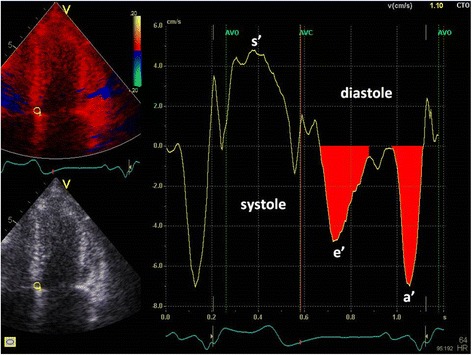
Image of colour tissue Doppler imaging with septal longitudinal displacement measurements depicted as area under the curve in early and late diastole. *s’, peak mitral annular systolic velocity; e’, peak early mitral annular diastolic velocity; a’, peak atrial mitral annular diastolic velocity; AVO, aortic valve opening; AVC, aortic valve closing*
